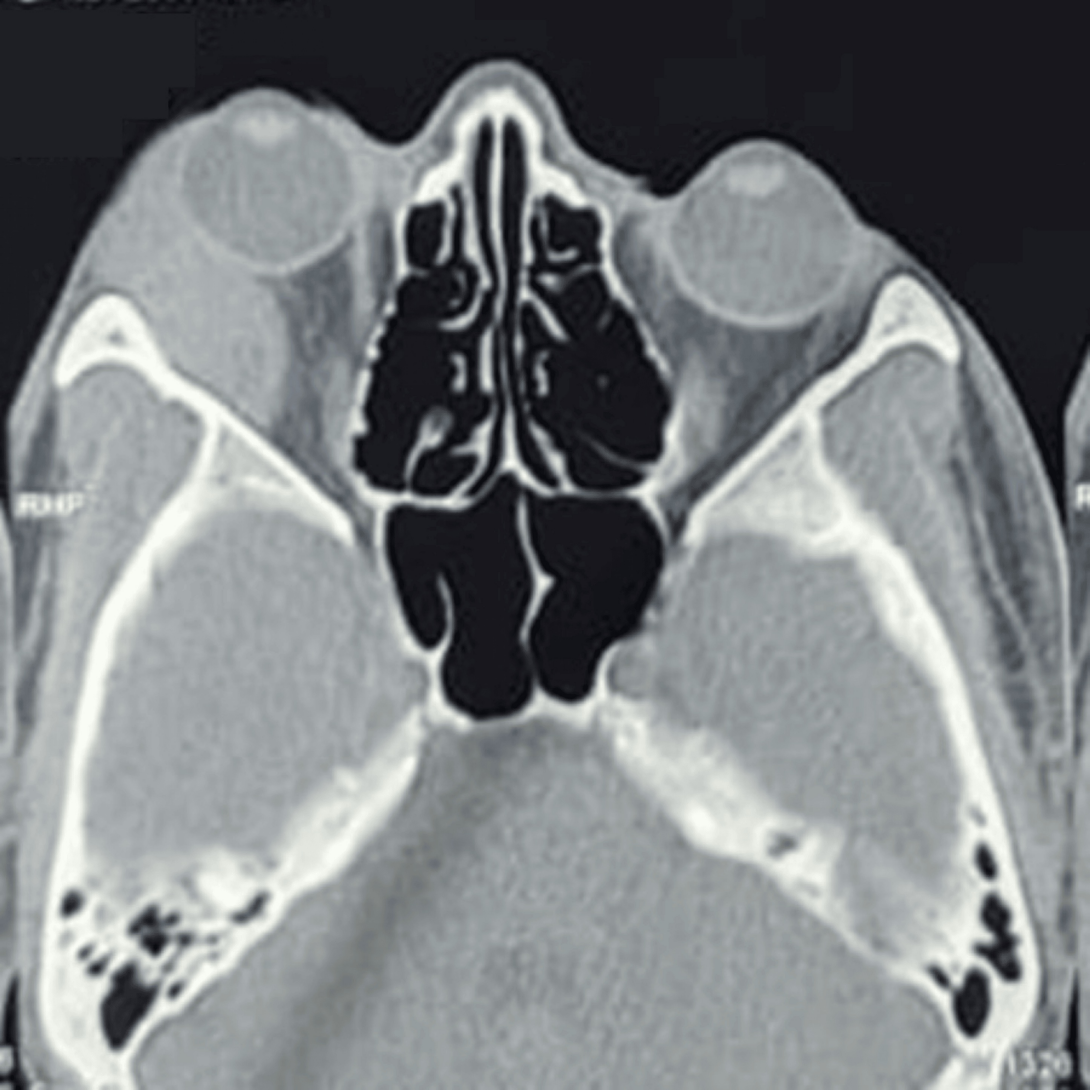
NCCT scan showing a soft tissue isodense lesion of size 33 x 12.2 x 33.5 mm3 in extra-conal space of right orbit on lateral aspect NCCT: Non-contrast computed tomography
